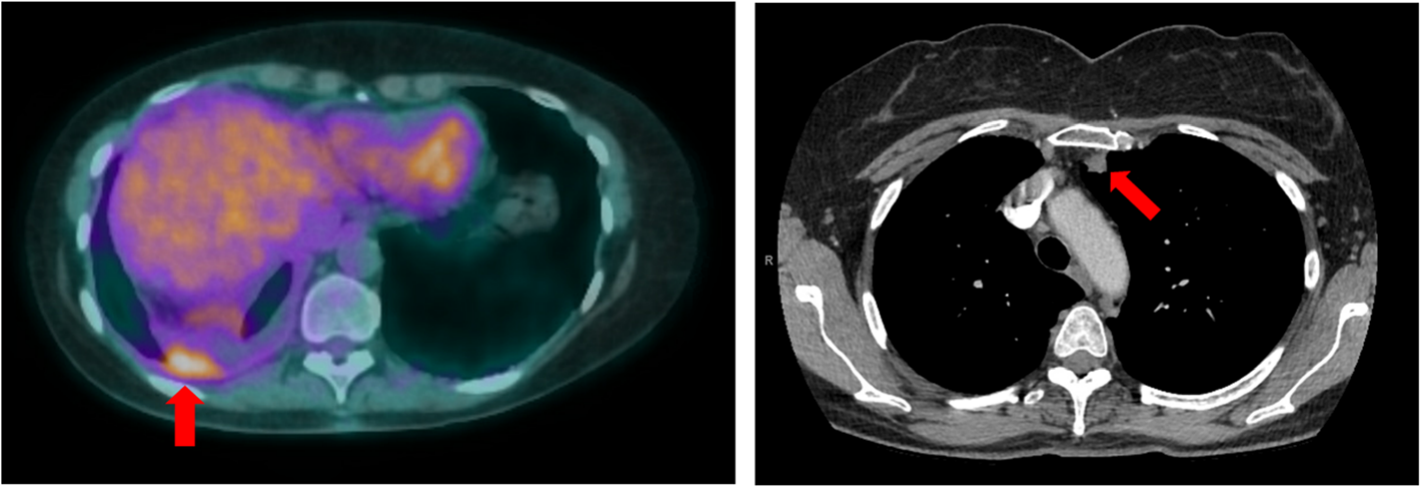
Sites of disease on recurrence. Pleural thickening with metabolic activity on the right was biopsied and pathology review confirmed mucinous adenocarcinoma with predominant lepidic pattern. In 3 months following radiation therapy of the recurring pleural lesion, there were new lung nodules identified on CT scan. One of these nodules is shown at the left upper lobe on the right
